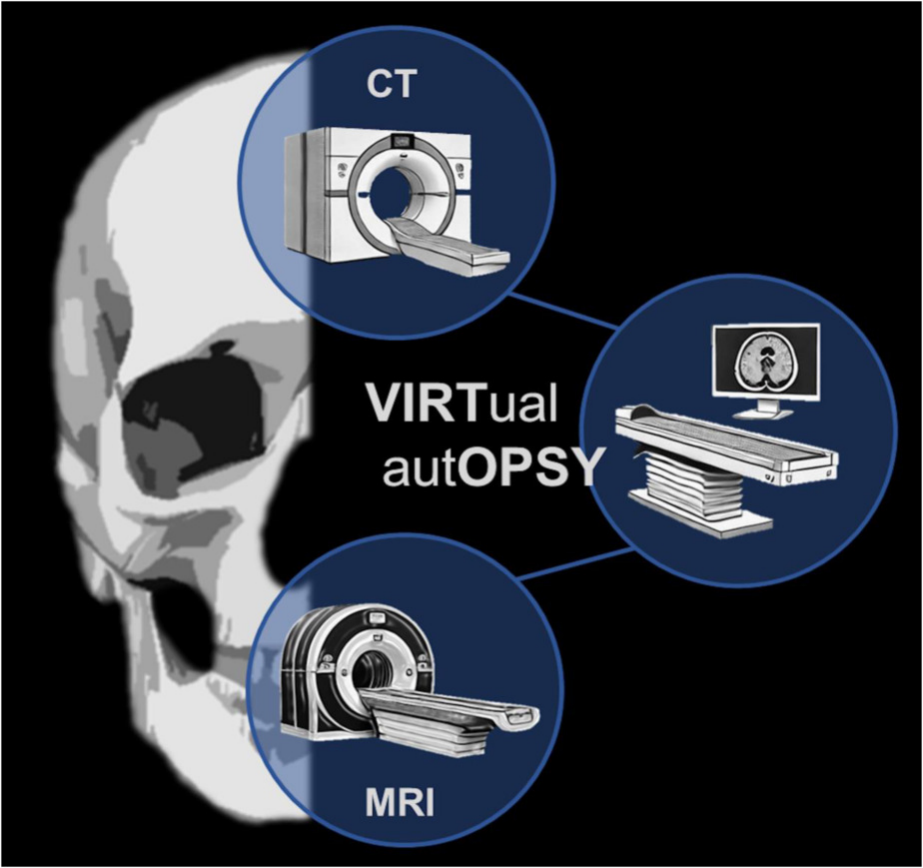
The term Virtopsy® (virtual autopsy) refers to the application of imaging technologies, such as CT and MRI, in forensic medicine to advance forensic death investigations. These post-mortem imaging techniques enable three-dimensional documentation of the entire body or specific regions, supporting accurate reconstructions and precise measurements.
